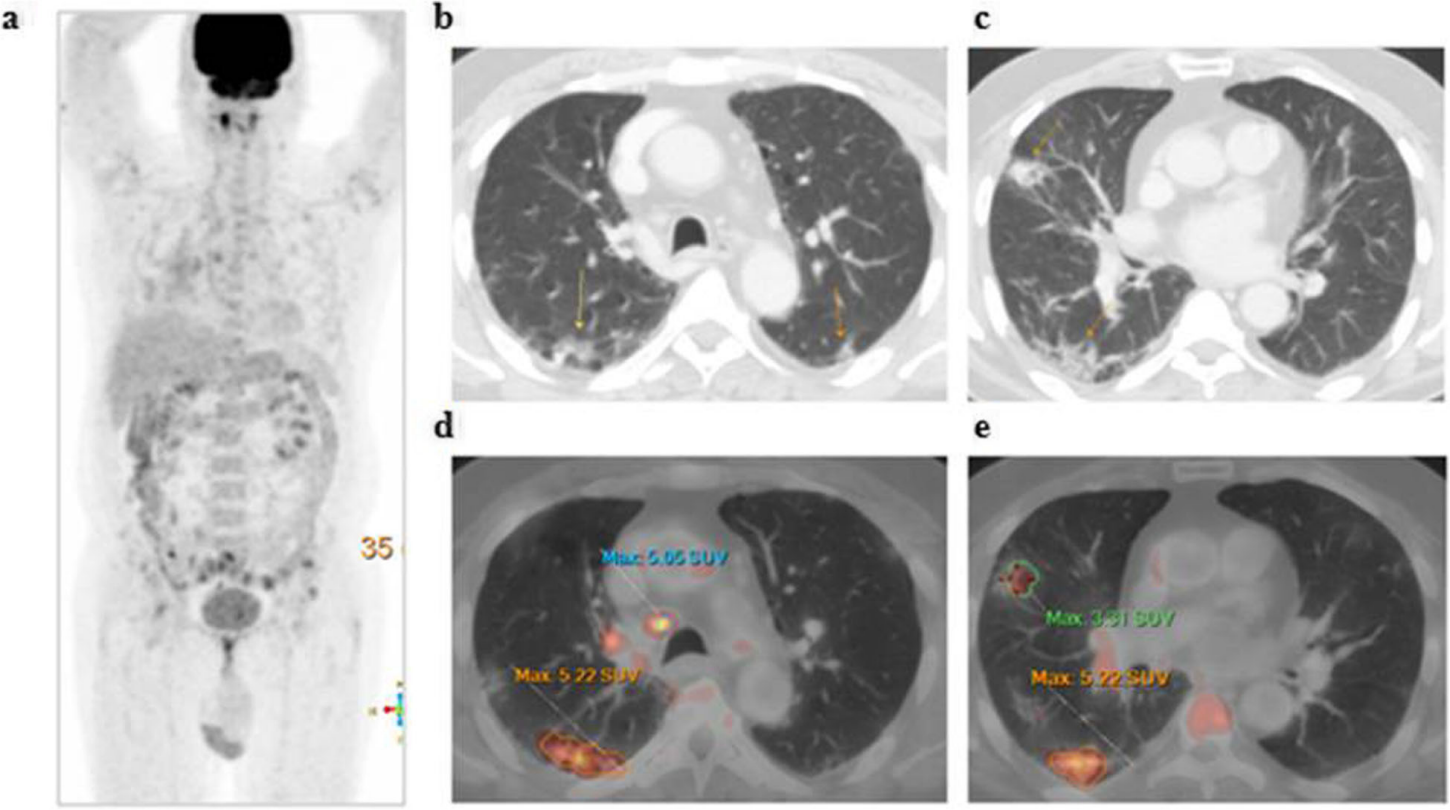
A 62-year-old male patient, with a history of surgically resected rectal cancer and referred for PET/CT follow-up. The whole-body MIP image (a) shows bilateral pulmonary metabolically active areas. Axial lung window CT images (b, c) revealed bilateral subpleural variable-sized ground-glass opacities with consolidation that show variable FDG avidity on corresponding fused PET/CT images (d, e) achieving up to 5.2 SUVmax
